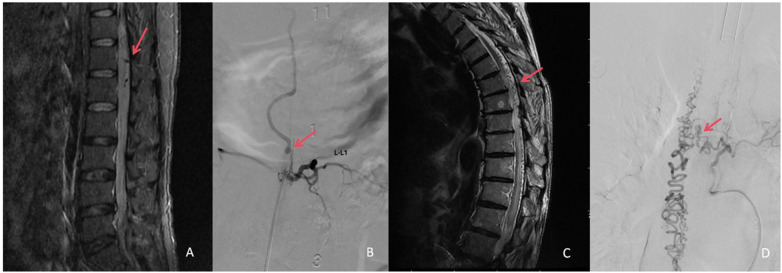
The images depict the MRI and angiographic findings of two patients. (A) The sagittal T2-weighted MRI of the first case reveals prominent perimedullary flow voids in the thoracolumbar region. (B) The results of digital subtraction angiography (DSA) for the first case, with the fistulous point indicated by an arrow. (C) The sagittal T2-weighted MRI of the second case demonstrates extensive perimedullary flow voids consistent with venous congestion within the thoracic spinal cord. (D) The DSA findings of the second case reveal dilated tortuous perimedullary veins (the venous drainage of the fistula).
